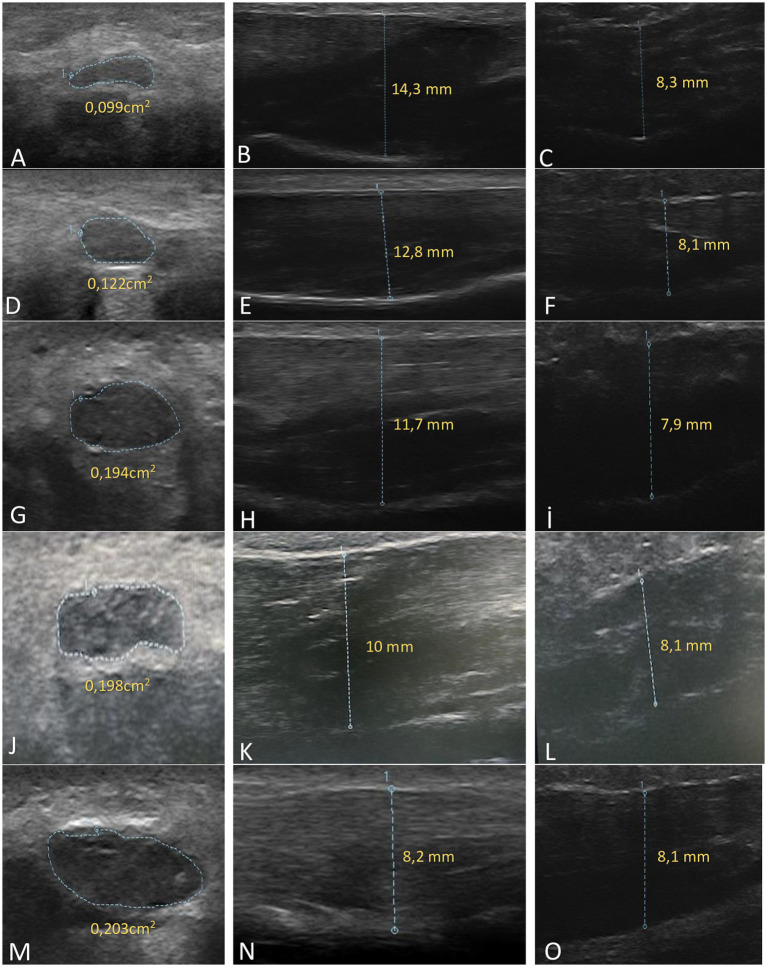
Ultrasonographic images demonstrating median nerve cross-sectional area, thenar muscle thickness, and hypothenar muscle thickness across different stages of carpal tunnel syndrome: minimal stage (A–C), mild stage (D–F), moderate stage (G–I), severe stage (J–L), and extreme stage (M–O).
